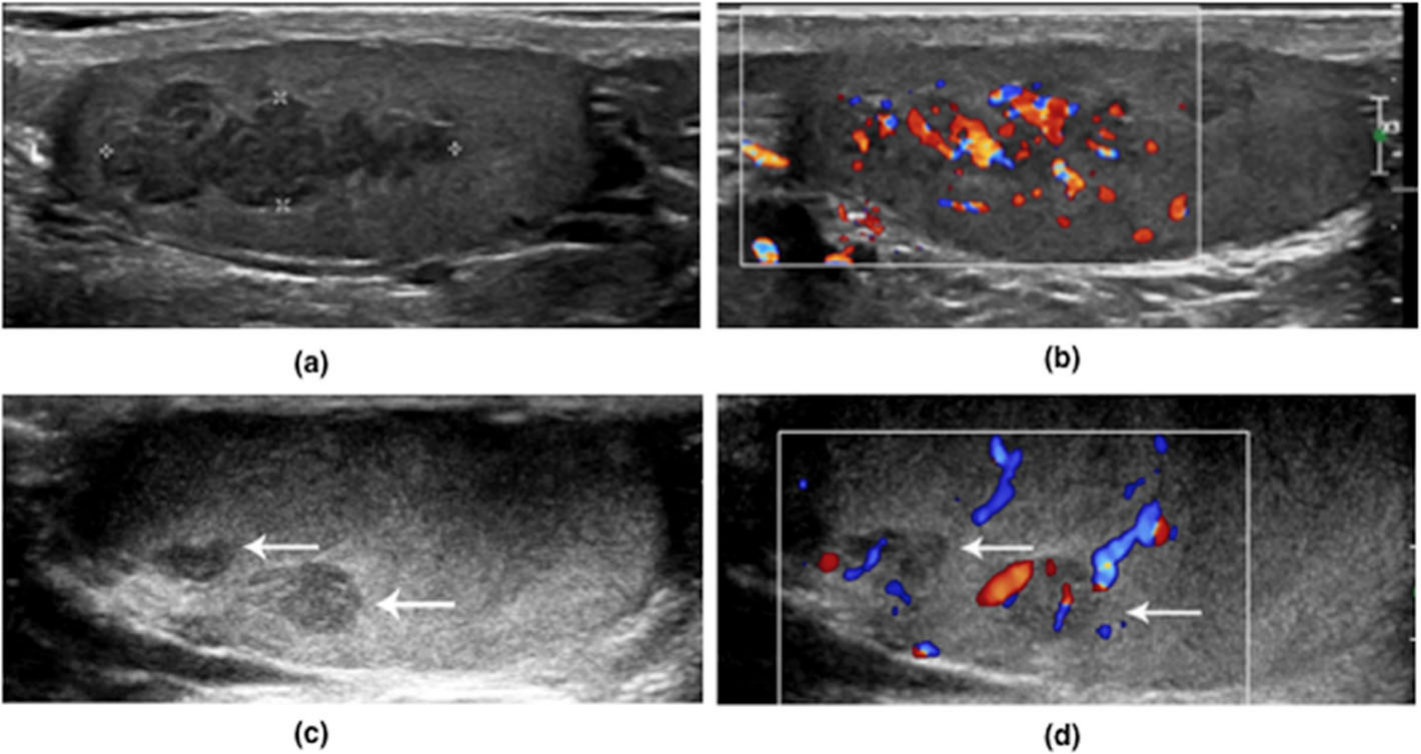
Ultrasound features of TART. This is a 13-year-old CAH patient. Palpable testicular nodules were detected through physical examination and a scrotal ultrasound was therefore performed. The scrotal gray-scale ultrasound (a) and color Doppler ultrasound (b) showed homogeneous hypoechoic lesions with clear boundary on both testes with marked vascularity. Follow-up gray-scale (c) and color Doppler (d) ultrasound examination was performed after a 6-month steroid treatment, which showed a remarkable decrease in lesion size and vascularity
